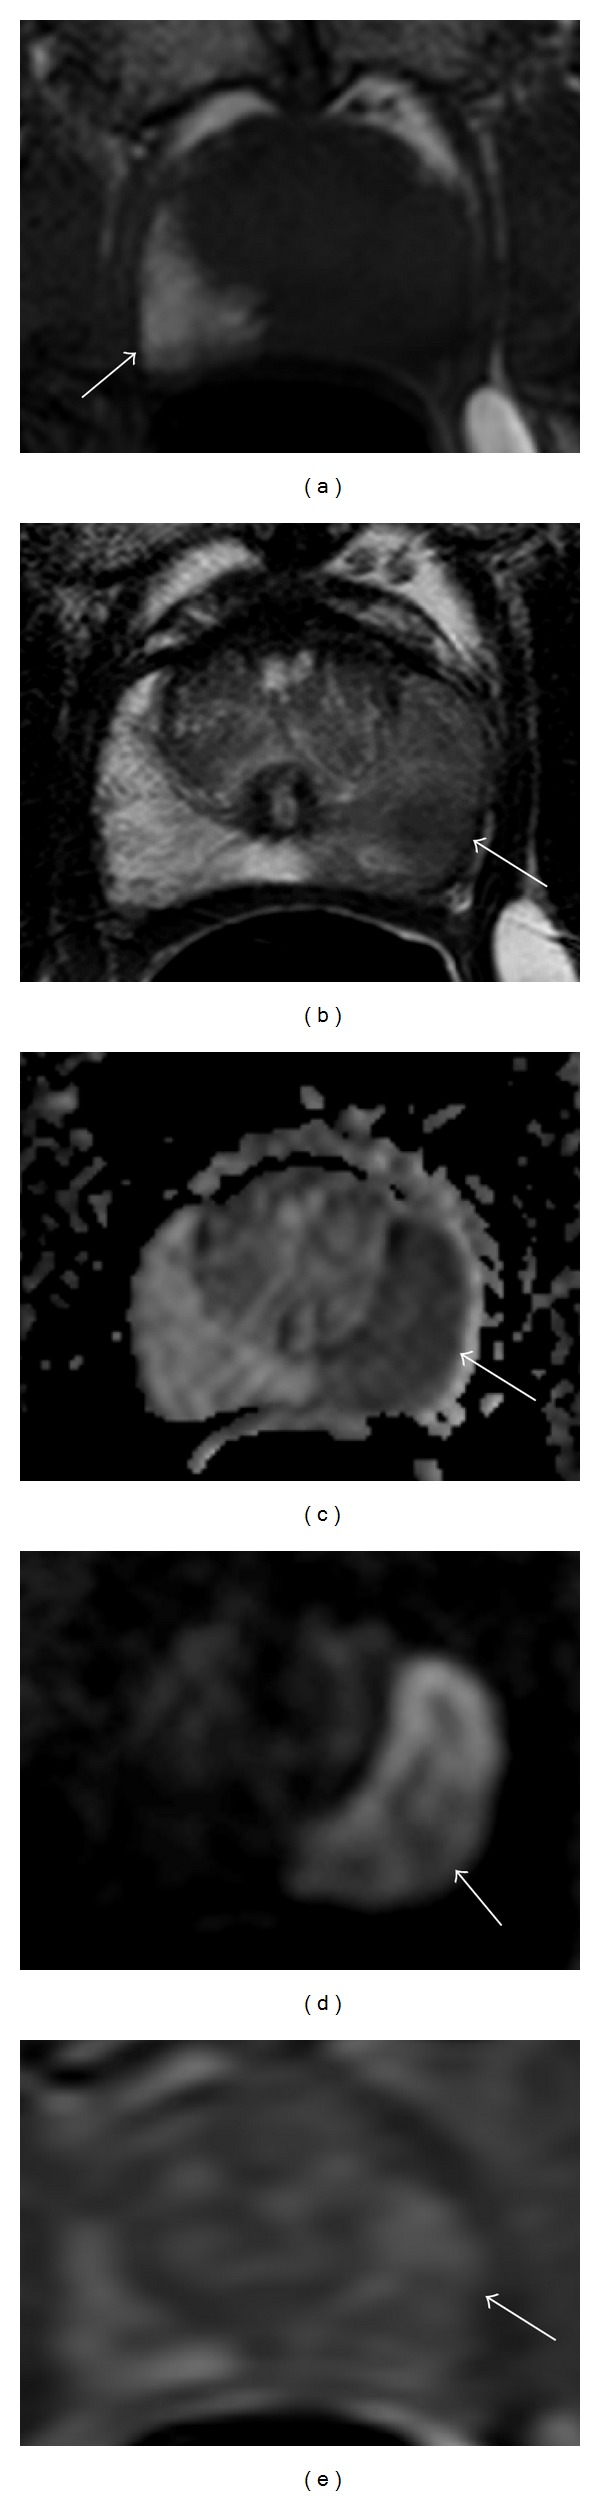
A 57-year-old patient, with PSA of 5.80, Gleason 4 + 5 on multiple cores, undergoing postbiopsy staging MP-MRI. Axial T1 weighted MRI shows biopsy related residual hemorrhage in right midperipheral zone (arrow) (a). Axial T2 weighted MRI shows a large left midperipheral zone lesion (b). Axial diffusion weighted MRI showing a large lesion extending throughout the left midperipheral zone (arrow) (c). High “*b*” value DWI (*b* = 2000) showing strong enhancement of the left midperipheral zone lesion (arrow) (d). Axial dynamic contrast enhanced imaging showing early enhancement in the left midperipheral zone (arrow) (e).
